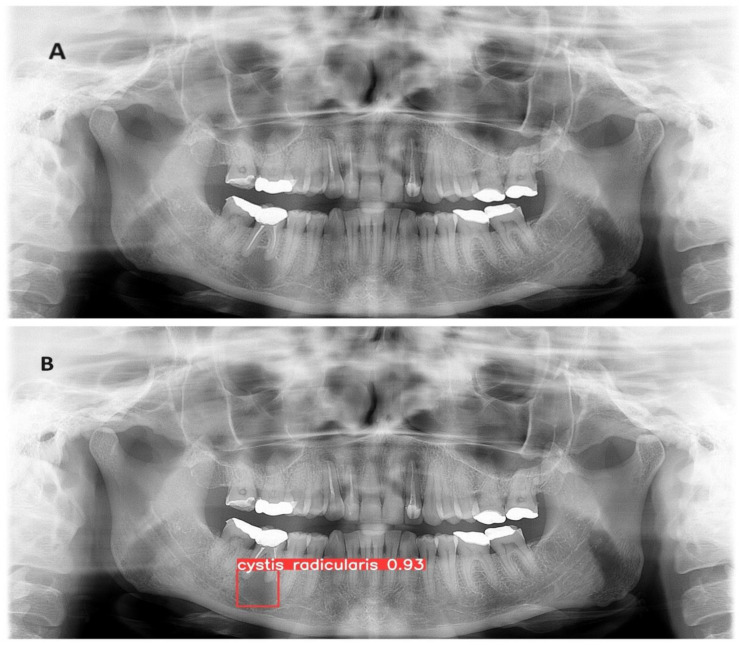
(A) Panoramic radiograph of radicular cyst in the lower jaw (B) Developed model detection prediction of a radicular cyst with precision of 93%.
